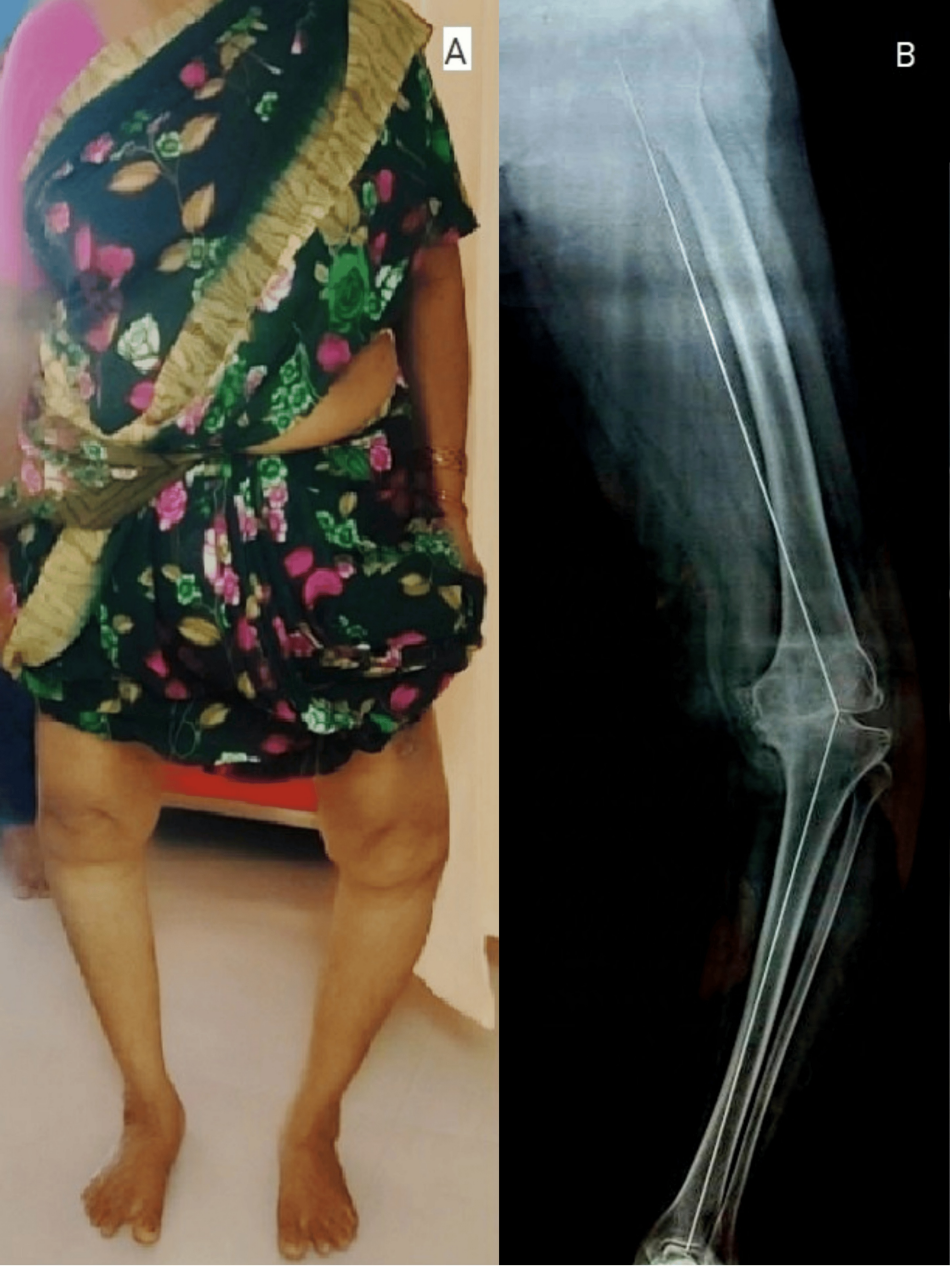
Preoperative clinical image (A) and full leg weight-bearing radiograph of the left lower limb (B) showing severe knee varus deformity.
